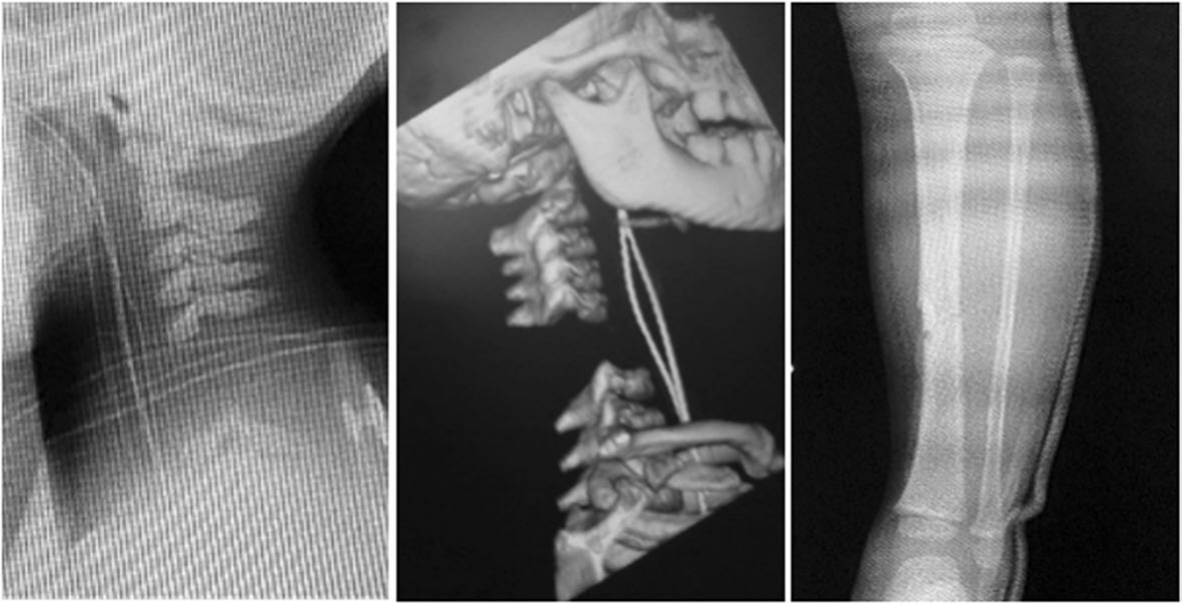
X-ray and CT images at the time of injury
